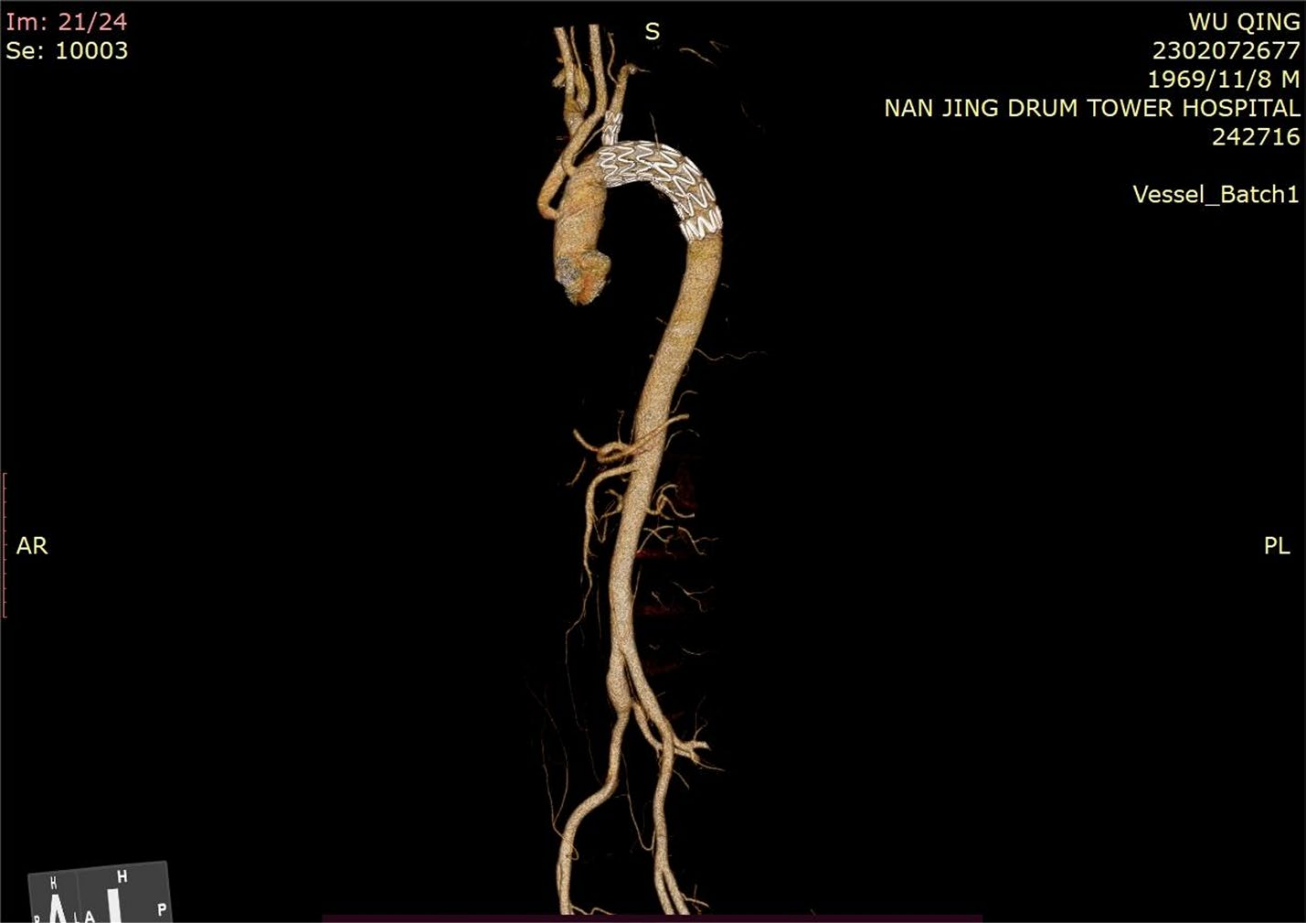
The results of the computed tomography angiography (CTA) follow-up after 3 months of Fontus stent implantation in the aorta
